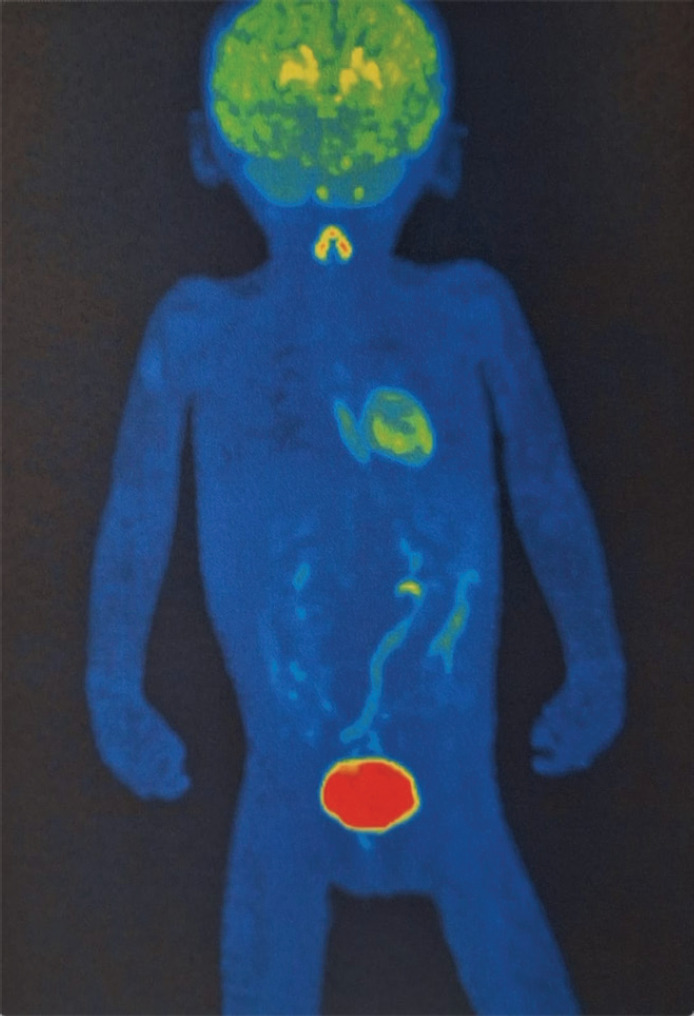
PET/CT FDG: Where the usual biodistribution of the radiotracer is identified in the cerebral cortex, basal ganglia, Waldeyer's ring, ventricular wall, thyroid gland, adrenal glands, kidneys, liver, spleen, intestinal tract and lungs, with elimination via the urinary route. No evidence of morphological or metabolic alterations. Free of gross tumour activity. Source: Medical registry.
